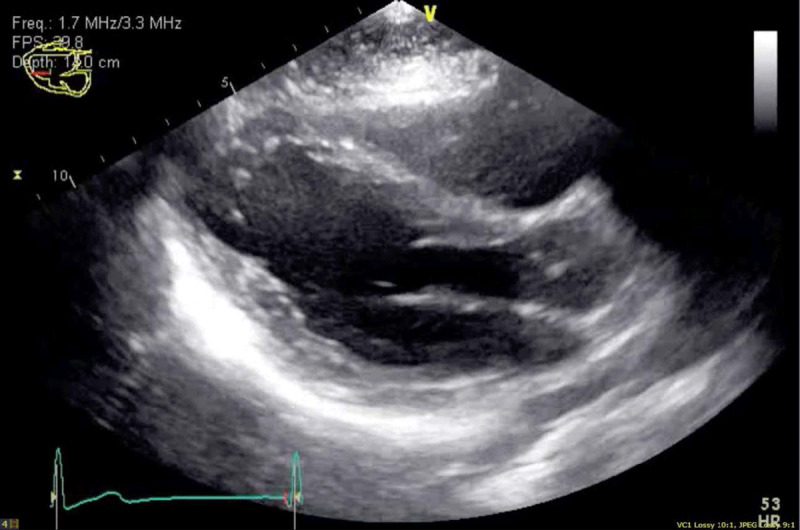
Transthoracic echocardiogram with a parasternal long-axis view that demonstrates a preserved left ventricular ejection fraction with no pericardial effusion.
